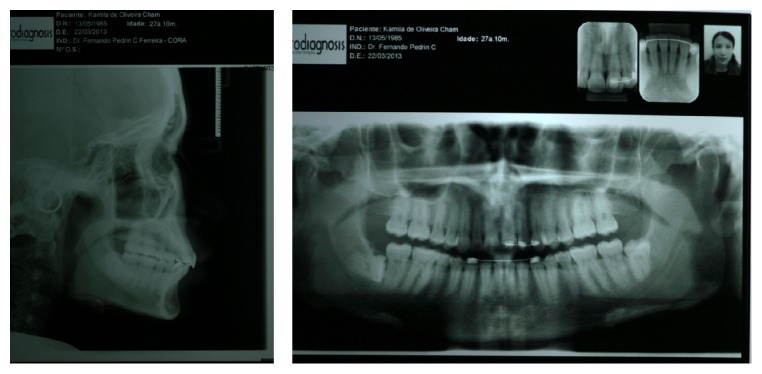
Final panoramic radiography and teleradiography. There was no significant root resorption.
